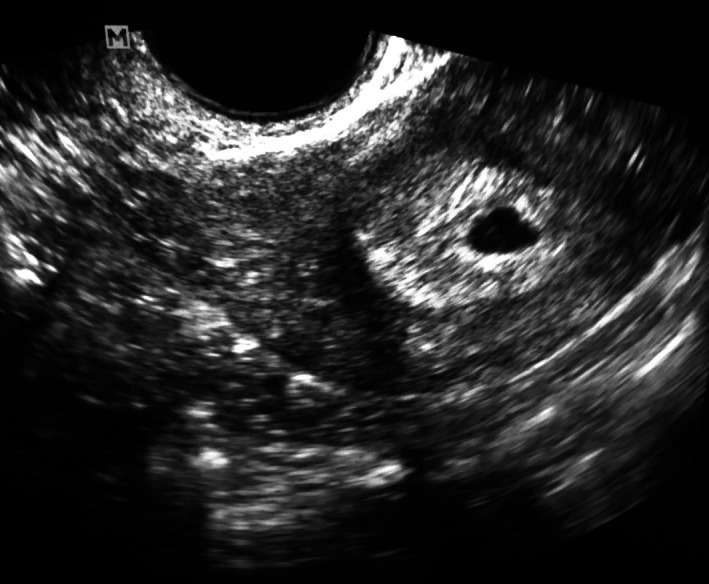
Pelvic ultrasound examination of the patient showing a fetus of 6 wk of pregnancy
